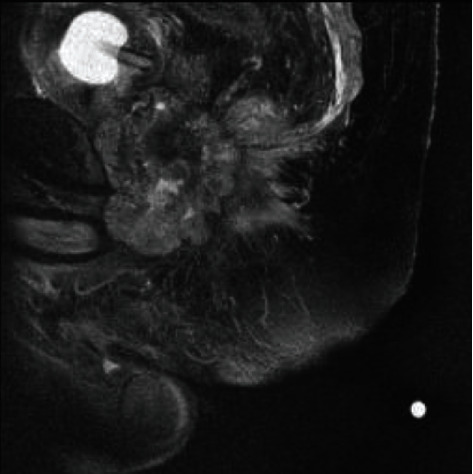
Saggital MRI imaging demonstrating persistence of collection post-antibiotic treatment.
